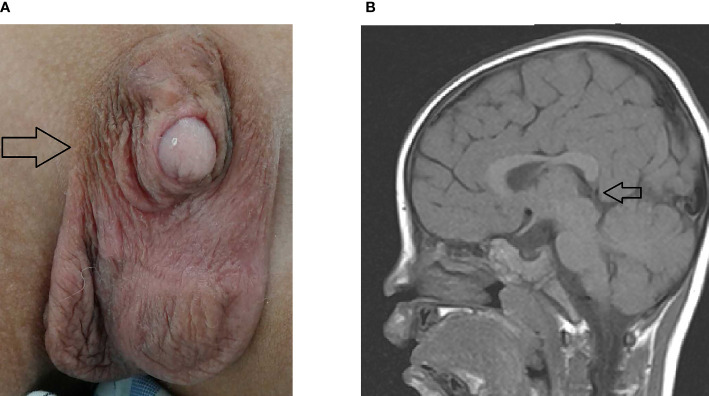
Patient 4 with hypocalcemic seizures that started 6 days after birth, had facial dysmorphism and hearing impairments. The patient’s calcium level was 6.0 mg/dL (ion calcium, 0.63 mg/dL). (A) He had ambiguous genitalia (arrow), scrotal bifida with hypospadias, penoscrotal transposition, congenital heart disease with double outlet right ventricle, ventricular septal defect, atrial septal defect, and pulmonary stenosis. Chromosome study confirmed the ring chromosome 9 and Kleefstra syndrome. (B) His MRI exhibited hypoplasia of splenium part of corpus callosum (arrow).
